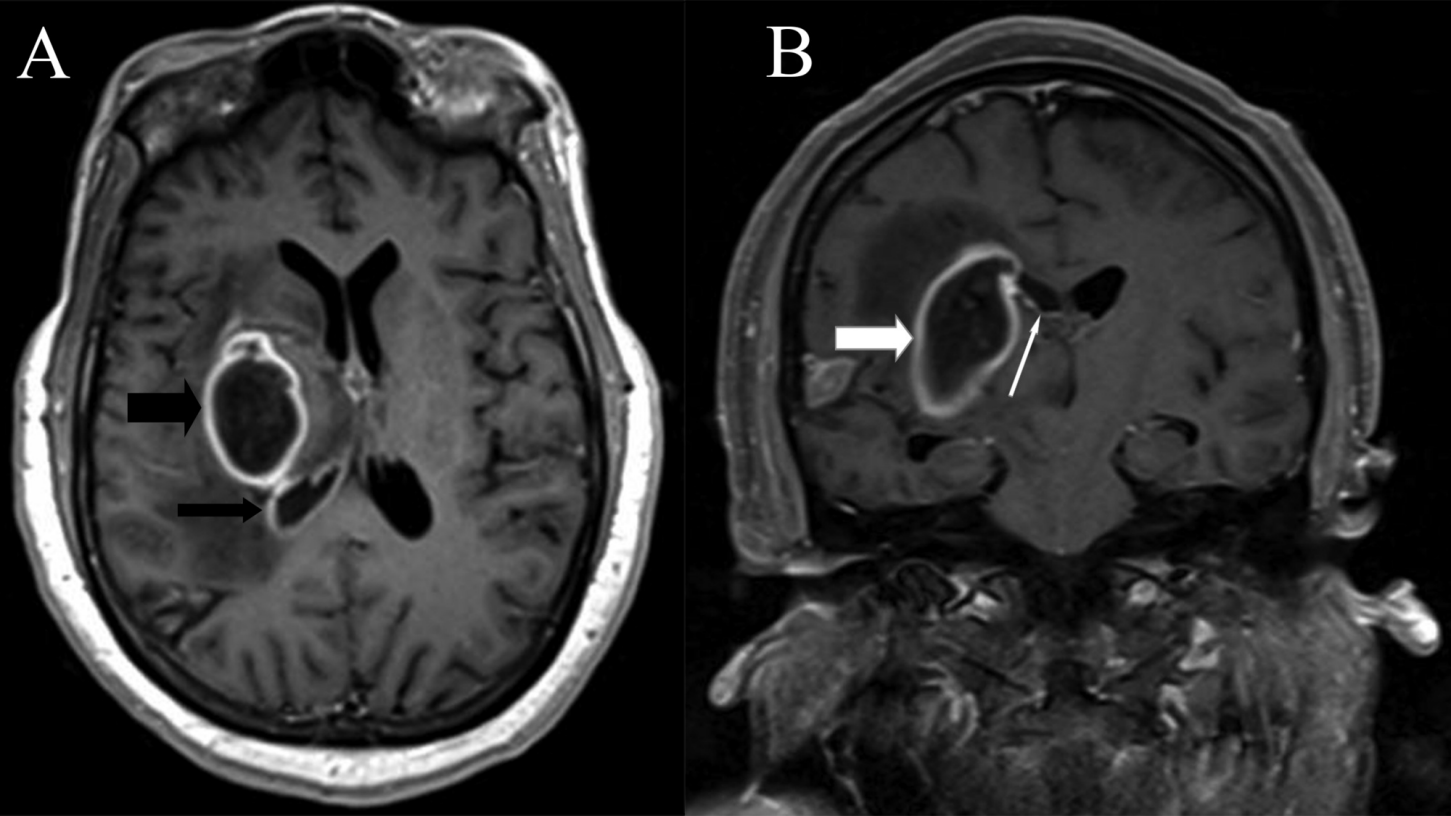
Repeat MRI after clinical deterioration A: Post-contrast T1 axial image demonstrating enhancement of the wall of the right lateral ventricle (thin black arrow) along with the previously described ring-enhancing lesion (thick black arrow). B: T1 post-contrast coronal section with ring-enhancing lesion (thick white arrow) and enhancement of the right ventricular wall (thin white arrow).
